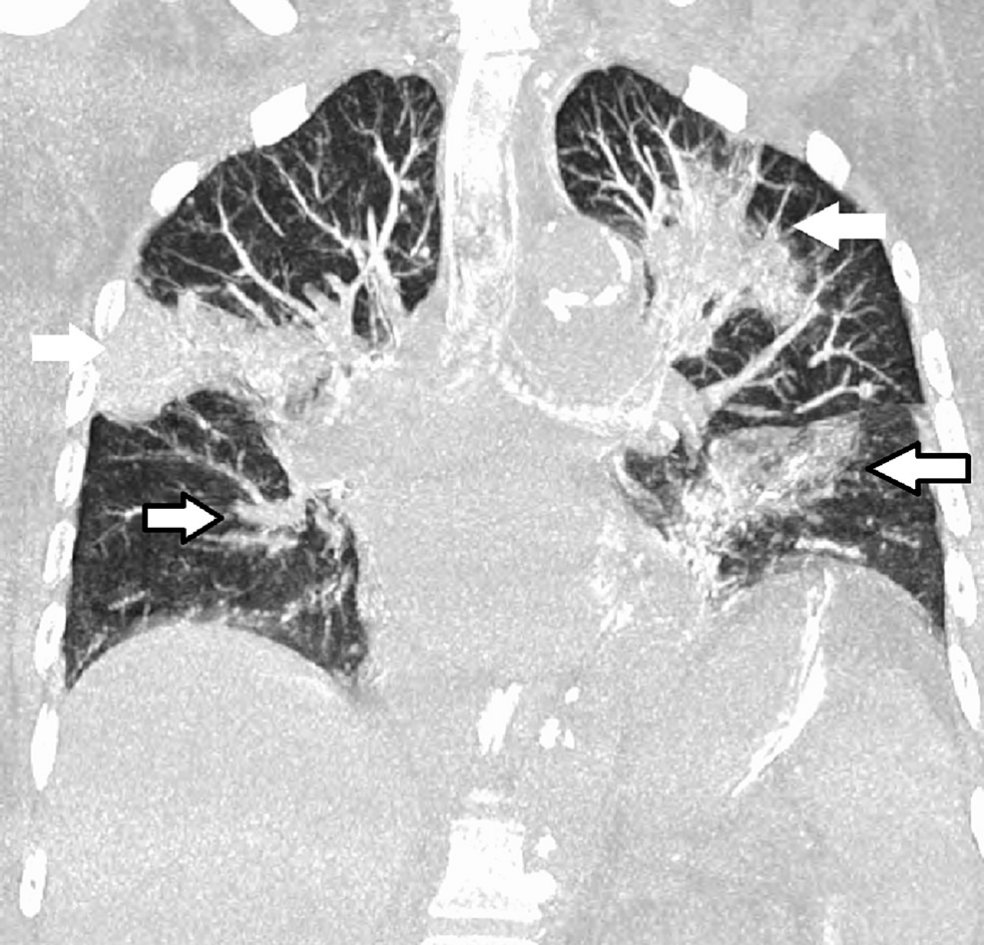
Coronal CT chest showing worsening bilateral infiltrates (white arrows) and new bilateral infiltrates (white arrows with black outline)
